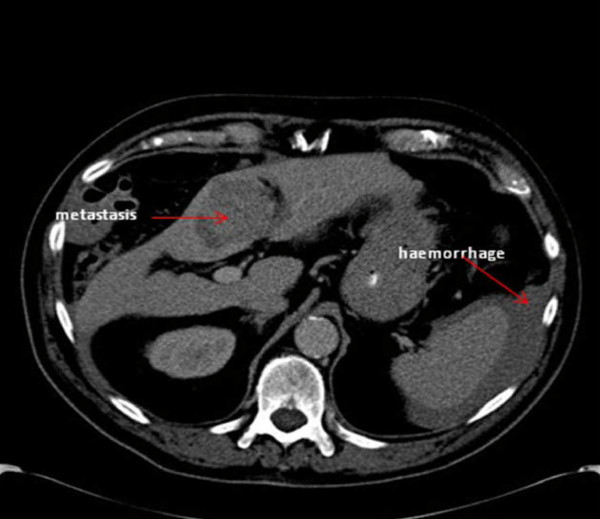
Axial computed tomography view of the patient showing metastasis and intra-abdominal hemorrhage (red arrows).
